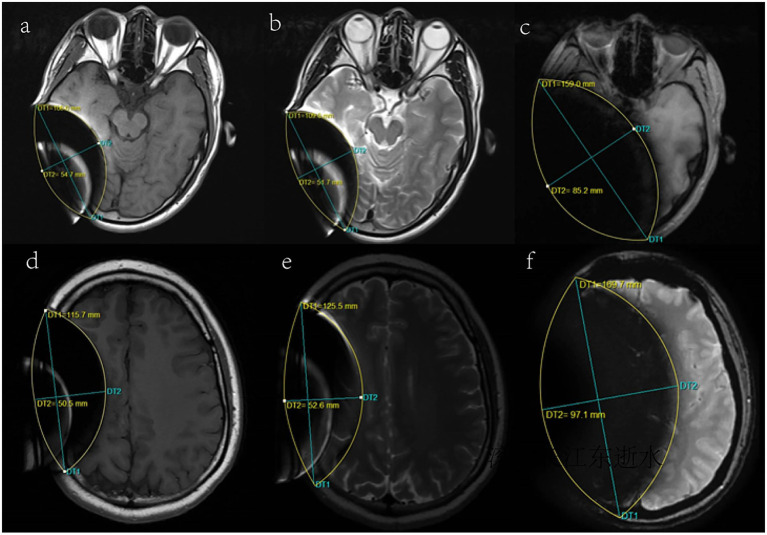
MRI artifacts across sequences in Volunteer 1 at 1.5 T and 3.0 T. (a) Signal void artifact on 1.5 T MRI (2D axial T1-weighted SE). (b) Signal void artifact on 1.5 T MRI (2D axial T2-weighted FSE). (c) Signal void artifact on 1.5 T MRI (2D axial T2-weighted GRE). (d) Signal void artifact on 3.0 T MRI (2D axial T1-weighted SE). (e) Signal void artifact on 3.0 T MRI (2D axial T2-weighted FSE). (f) Signal void artifact on 3.0 T MRI (2D axial T2-weighted GRE). The dashed lines indicate the two orthogonal measurements of the artifact (major and minor axes).
